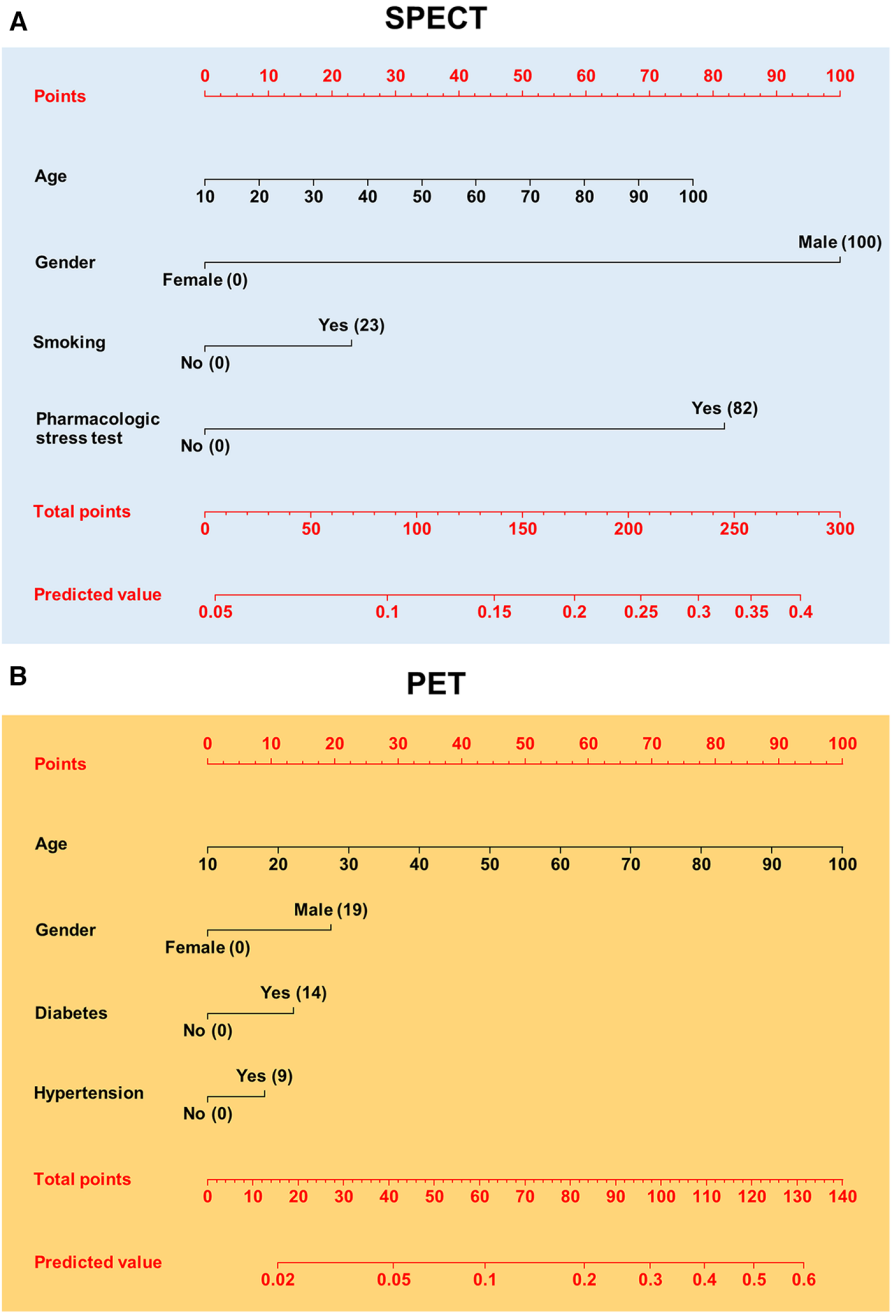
Nomograms for pretest calculation of patients to referral SPECT (A) or PET (B). The points related to risk factors appear in parentheses. Corresponding to total points, the predicted value for an abnormal MPI is given.
